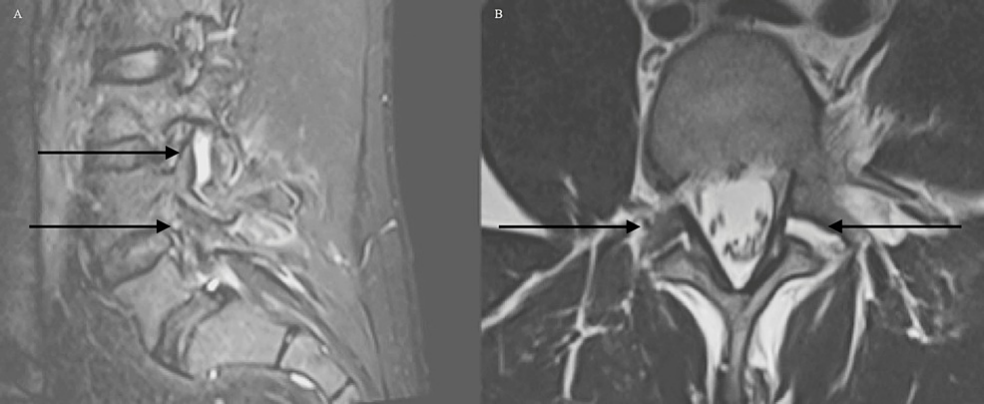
MRI of the lumbar spine A) Sagittal STIR sequence demonstrating widening of the left facet with evidence of facet capsule injury; B) axial T2 sequence demonstrating widening and edema of bilateral L4/5 facet joints left worse than right STIR: short tau inversion recovery
